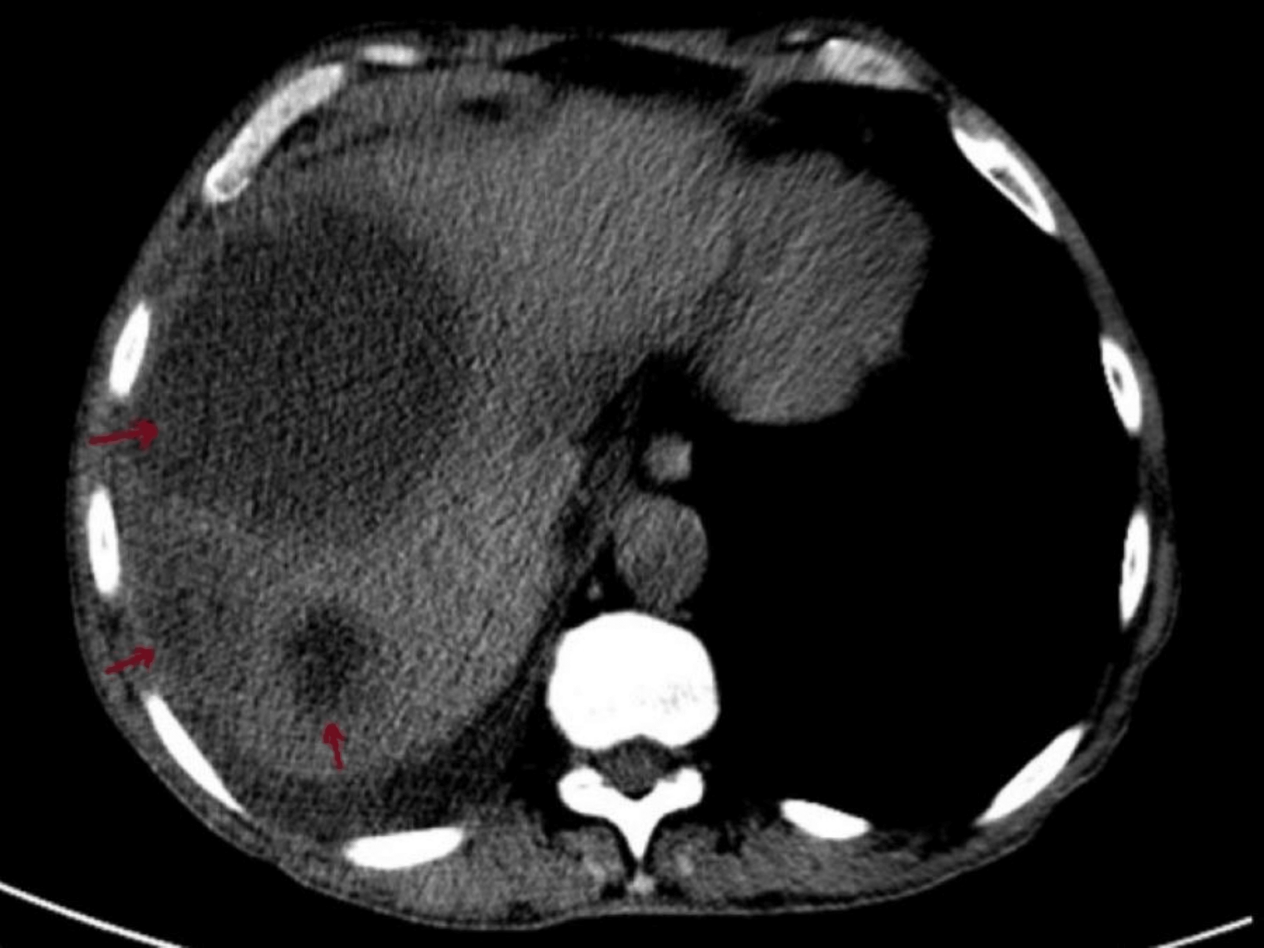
Abdominal CT scan showing nodular hepatic lesions (arrows).
